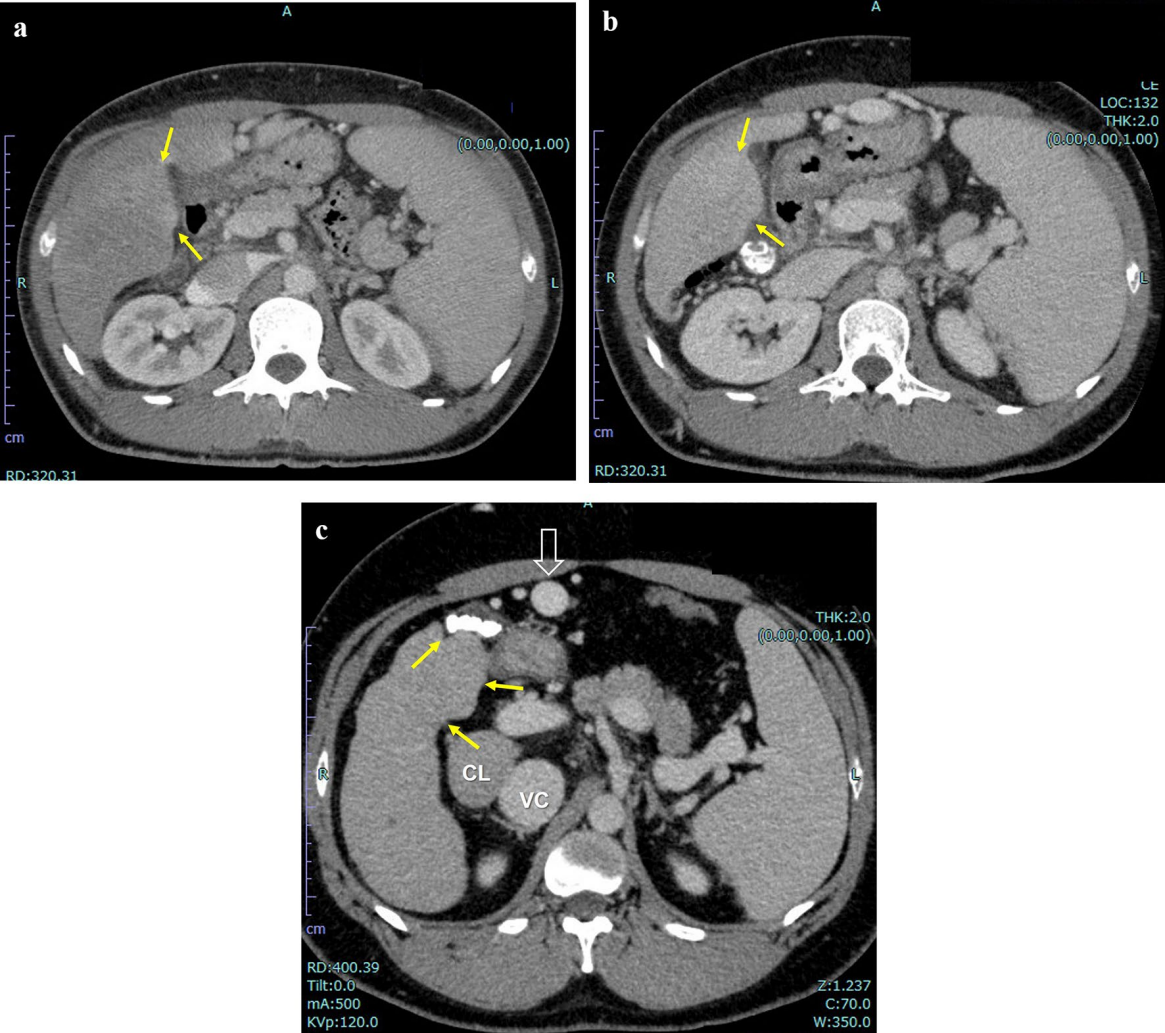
Longitudinal change of gallbladder fossa nodularity (GBFN) in 28-year-old man with alcoholic liver disease. a Portal phase of contrast-enhanced CT reveals hepatosplenomegaly and grade 1 GBFN (arrows). Clinically, this patient was in the acute phase of alcoholic liver injury at this time. ALBI score and modified ALBI grade were − 2.1 and grade 2b. b Portal phase of contrast-enhanced CT one year later again revealed grade 1 GBFN (arrows). ALBI score and modified ALBI grade were − 1.5 and grade 2b. c Portal phase of contrast enhanced CT 4 years later (5 years from 6a) revealed grade 3 GBFN (arrows). ALBI score and modified ALBI grade were − 1.4 and grade 2b, respectively, suggesting deterioration of liver function since 6a. Note enlarged caudate lobe (CL), irregular surface of the lateral aspect of the right lobe, enlarged paraumbilical vein (empty arrow), and enlarged inferior vena cava (vc) due to massive splenorenal shunt (not shown). Also note this slice is several cm cephalad to 6a or 6b, indicating marked atrophic change of background liver and progression of cirrhotic change
